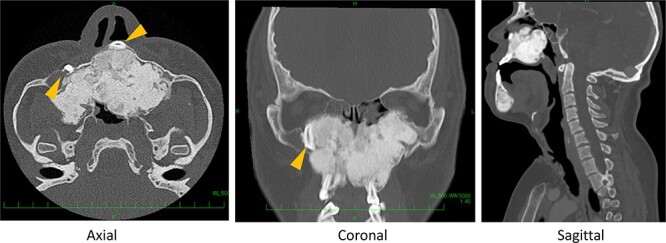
Preoperative computed tomography (CT) shows severe bone formation with inversed teeth (arrow heads); space between nose and pharynx was narrow.
